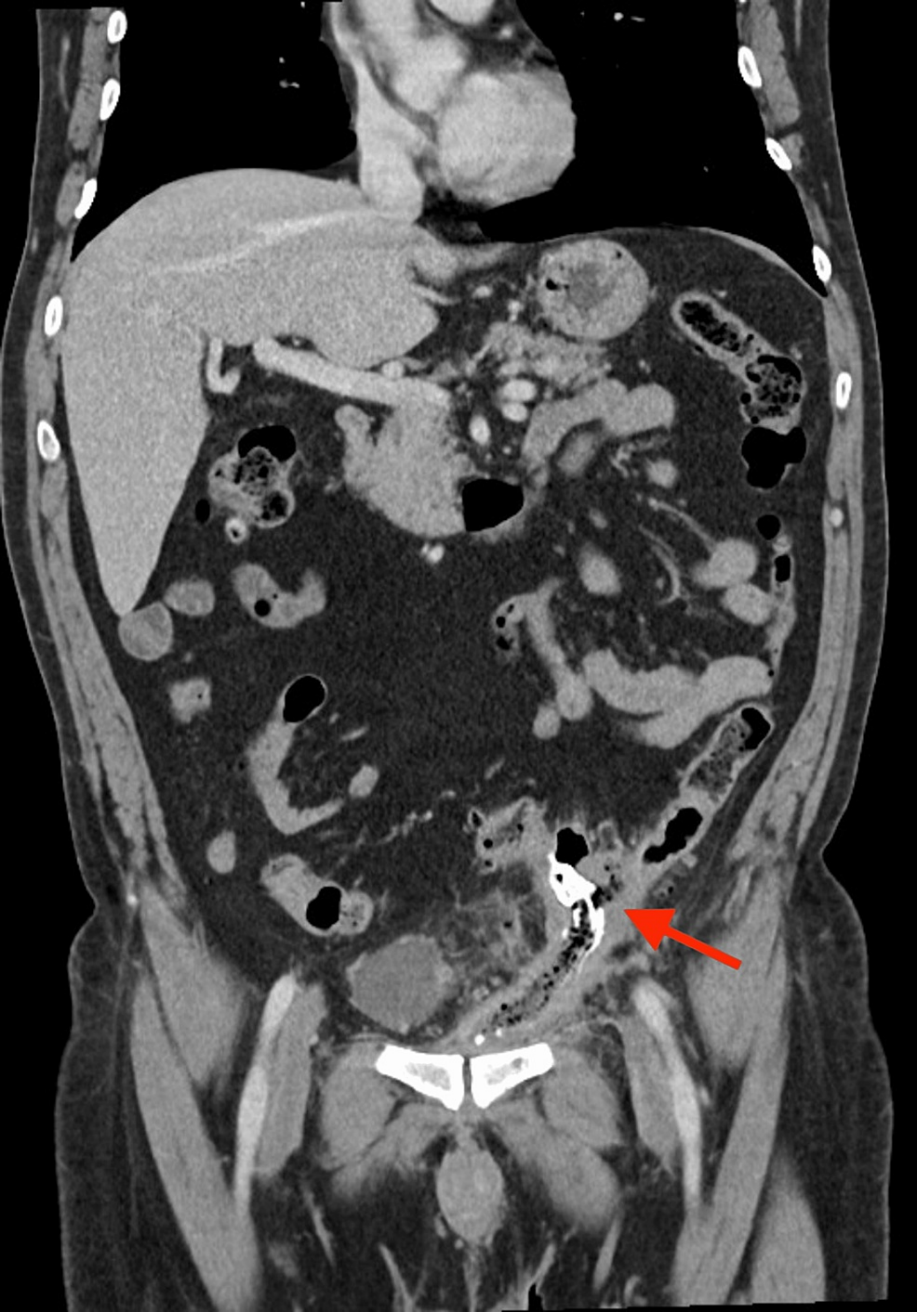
Abdominal CT scan (coronal plane) showing foreign body (arrow) partially in the colon lumen and intraperitoneal collection with air
